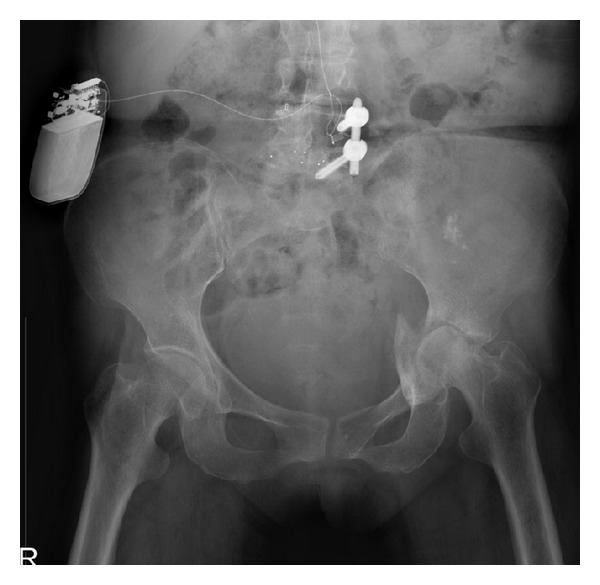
Radiograph taken 4 months after seizure episode showing left hip joint-space narrowing with femoral head destruction.
